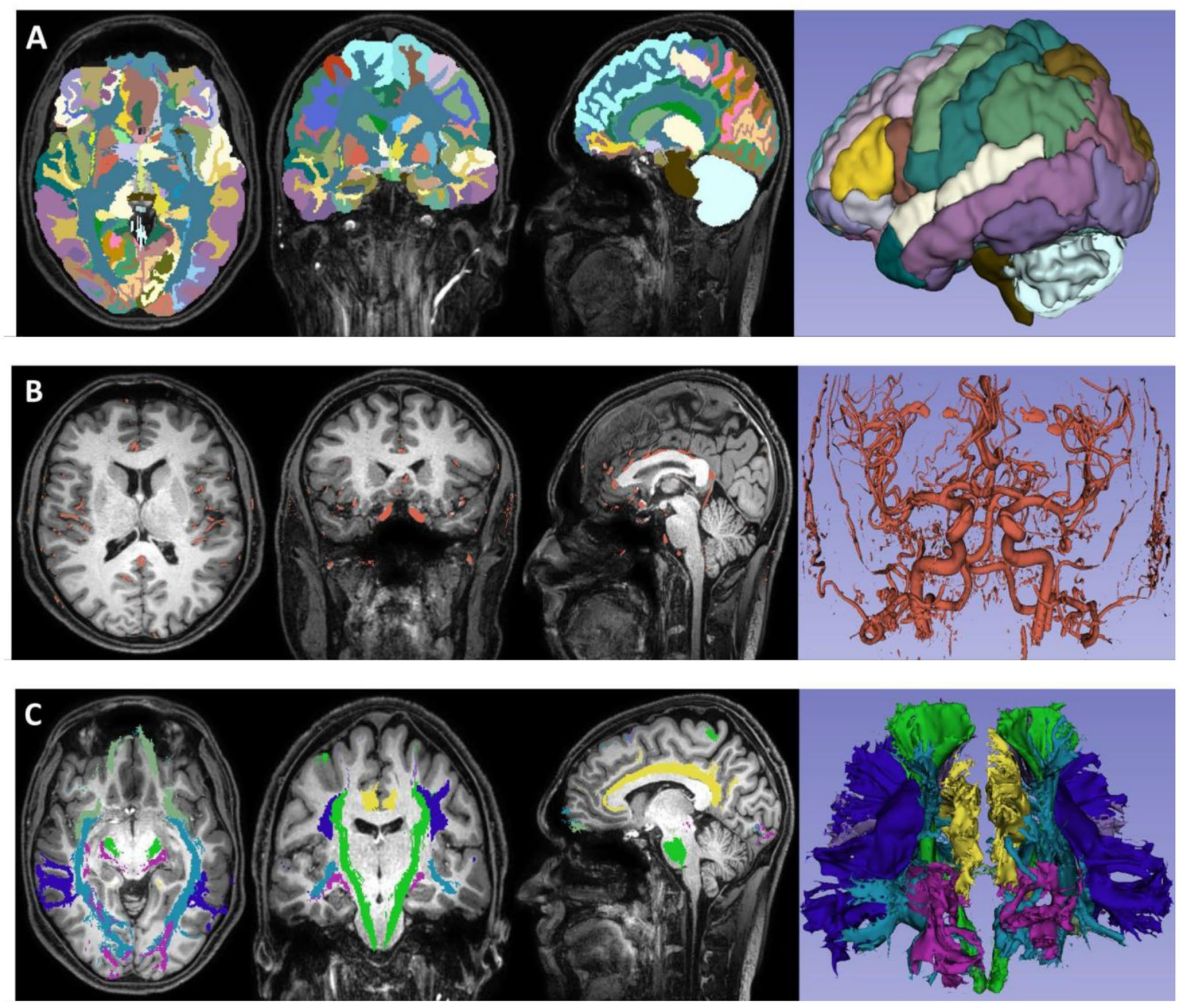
The extracted segments, vascular fields, and main tracts of the chosen sample from the cohort. This picture was obtained by segmenting the MRI images taken by the radiology department through the program. The anatomical areas in these MRI scans were evaluated using the coordinate system. (A) segmented brain image; (B) extraction of the vascular anatomy; (C) reconstruction of the main tracts
